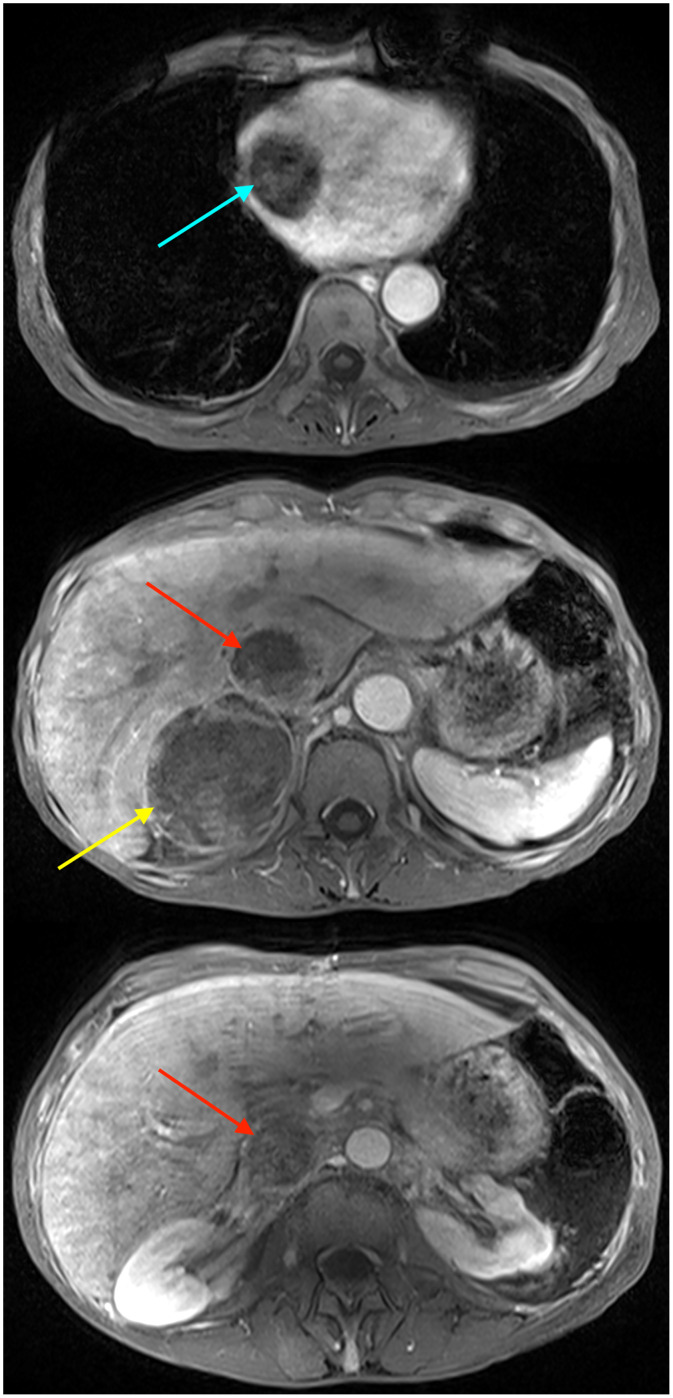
Magnetic resonance imaging of the abdomen with contrast (70 second delay) in axial view demonstrates a 8.2 cm right adrenal mass (yellow arrow) with intravascular tumor invasion into the inferior vena cava (red arrow) and right atrium (blue arrow).
